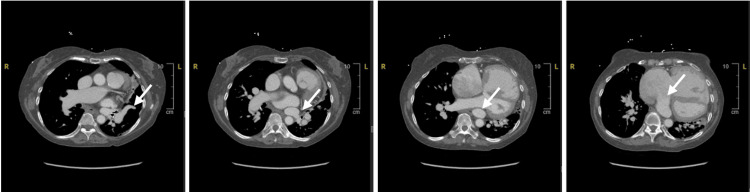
Cardiac CT of the left inferior pulmonary vein Cardiac CT demonstrating left inferior pulmonary venous drainage into the coronary sinus and ultimately into the RA (left to right). CT, computed tomography; RA, right atrium.
